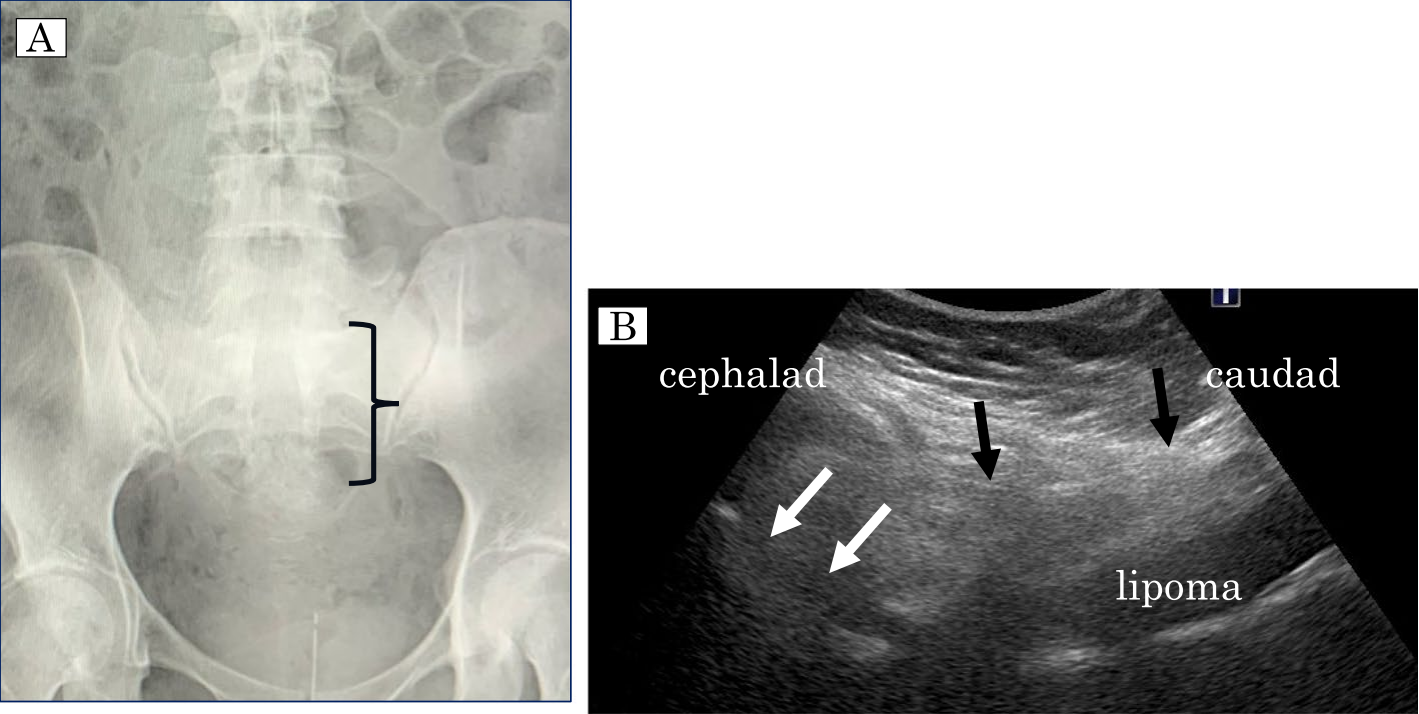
A Radiographical image of the lumbar spine on AP view. Large bone defects from L5 to the sacrum are indicated. B Ultrasonographic image of sagittal view from lower lumbar spine to sacrum. Large bone defects and lipoma beyond the bone defects are shown. The spinous process of the lower lumbar spine is hypoplastic and the vertebra fusion is incomplete. Two black arrows show the soft tissue continuity from the skin to the lipoma. Two white arrows show the cessation of tissue continuity from the lipoma to the cephalad soft tissue
